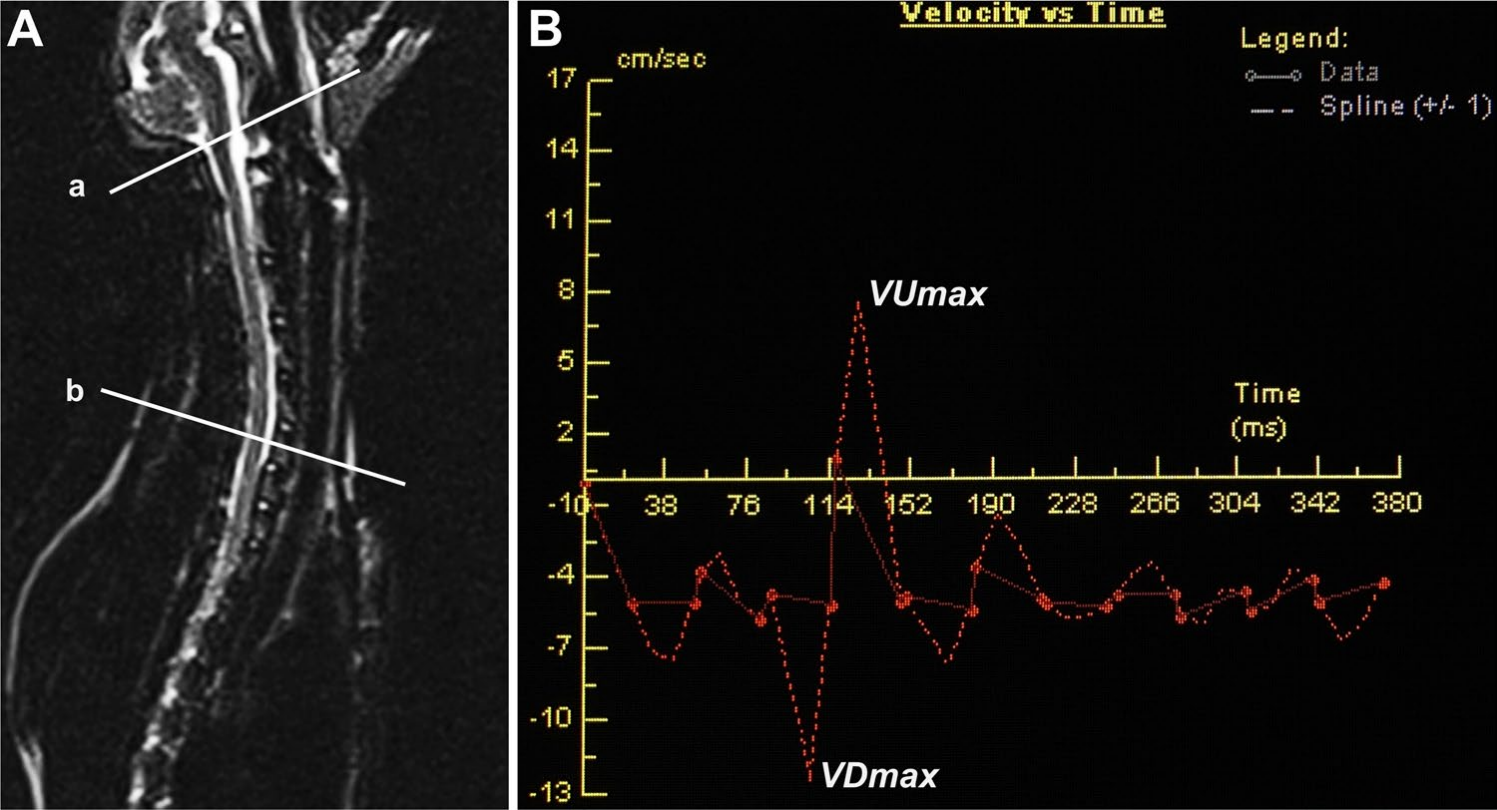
The measurement of CSF flow by PC-cine MRI observation. CSF flow was measured at C0 (line a) and C7 (line b) levels (A). CSF flow was analyzed by Argus postprocessing program, the peak velocities of CSF flow were measured on the basis of the CSF velocity-time curve, including up-flow (marked by VUmax) and down-flow (marked by VDmax) (B).
